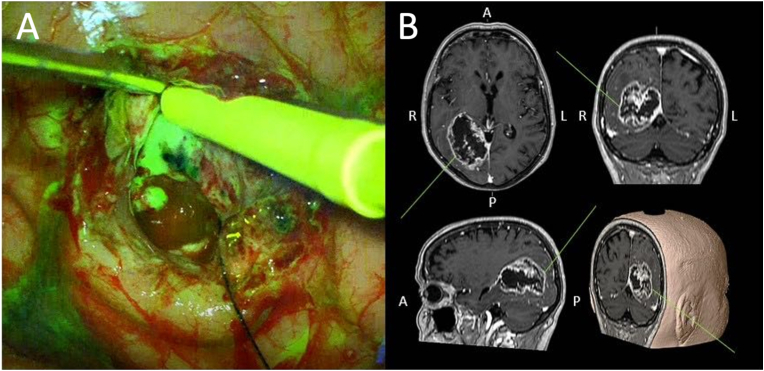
Resection of fluorescent glioblastoma tissue using an ultrasonic aspirator in a subcortical region (A), guided by axial, sagittal, coronal, and 3D-reconstructed MRI navigation (B).
